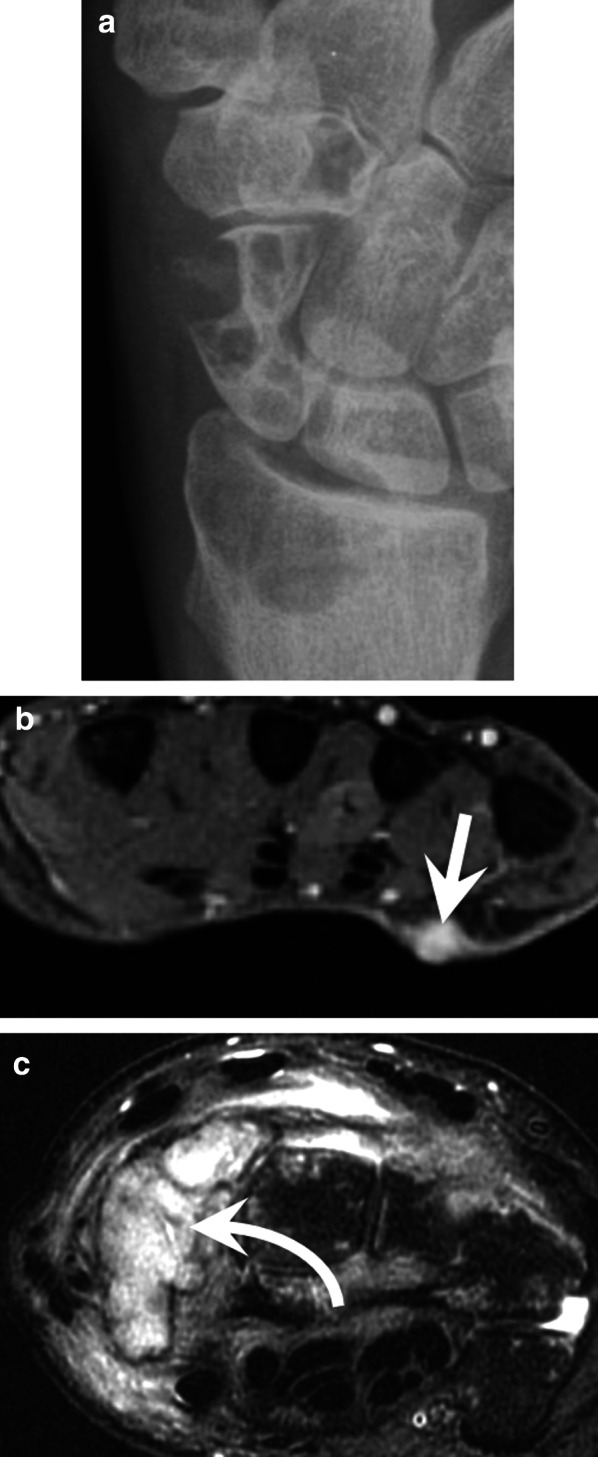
Epidemic Kaposi’s sarcoma in a 40-year-old man. (a) Standard radiography showing bone lytic tumours of the radius, scaphoid, and trapezoid bones. (b, c) Axial T2-weighted MR images showing a small cutaneous tumour of the palm (straight arrow), a tumour invading the scaphoid bone (curved arrow) and soft tissue infiltration
